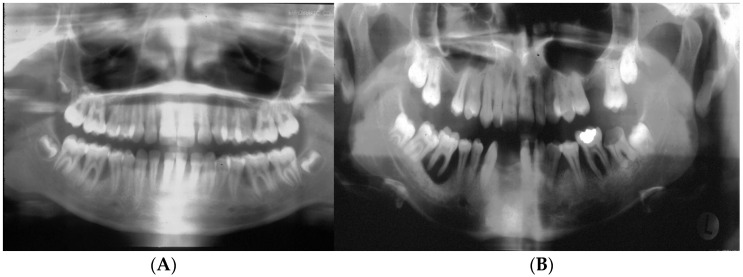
Panoramic radiographs of cases of aggressive periodontitis in adolescents. Panel (A) shows a panoramic view of excessive bone loss in the first molars and no carious lesions. Panel (B) shows more extensive disease in an adolescent with bone loss around the molars and missing molars and incisors with occlusal, but proximal decay is related to a blow-out occlusal lesion in the mandibular right second molar.
